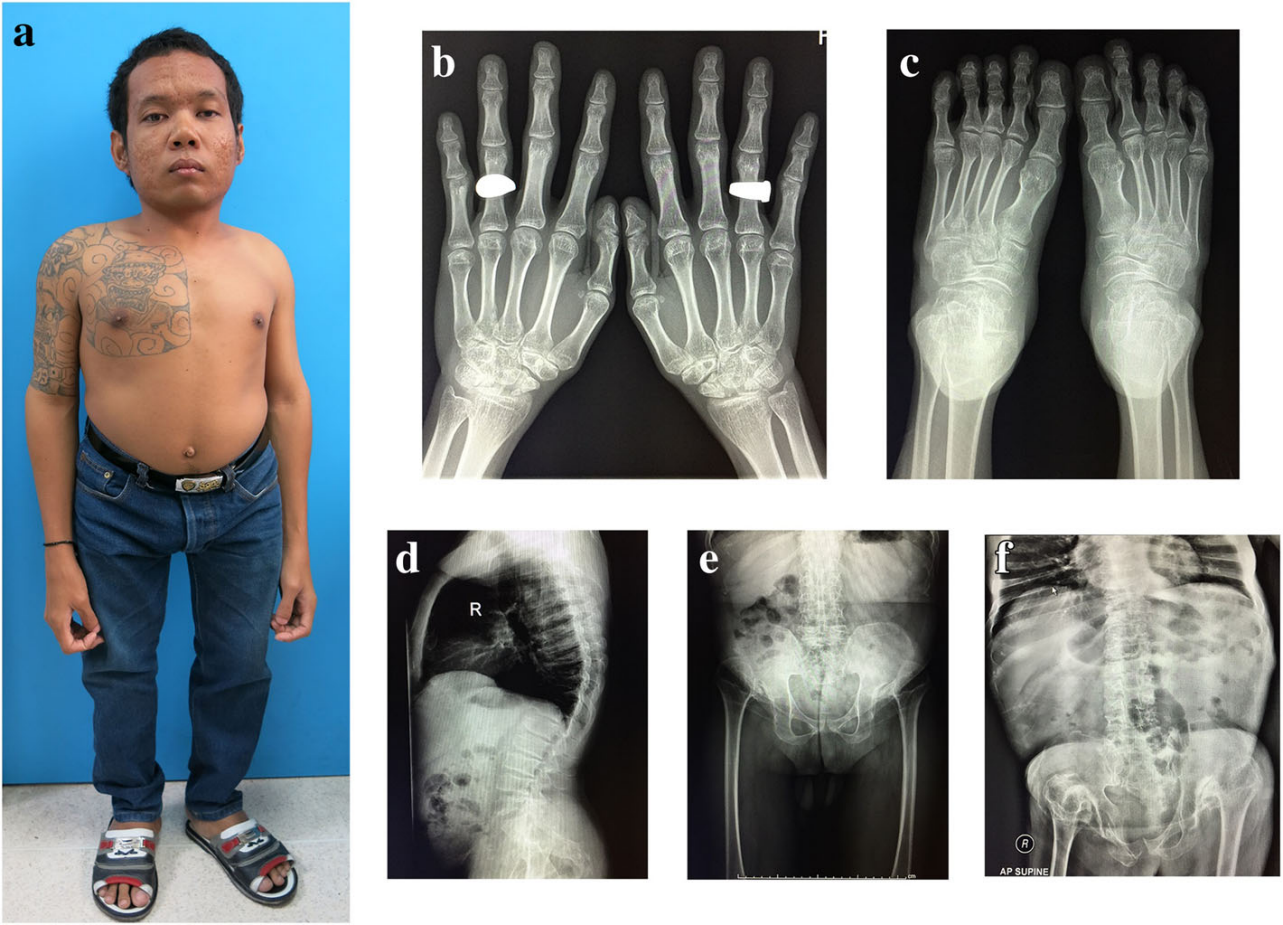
a Photograph of proband’s father (III: 1) showing disproportionate short stature and barrel-shaped chest. b, c Hand and feet radiographs are normal without brachydactyly. d Lateral radiograph of the thoracolumbar spine shows platyspondyly with kyphotic deformity. e Pelvis AP radiograph showing severe hip dysplasia and dislocation. f Thoracolumbar spine and pelvis AP radiographs of the proband’s grandfather also show platyspondyly and severe hip dysplasia and dislocation
